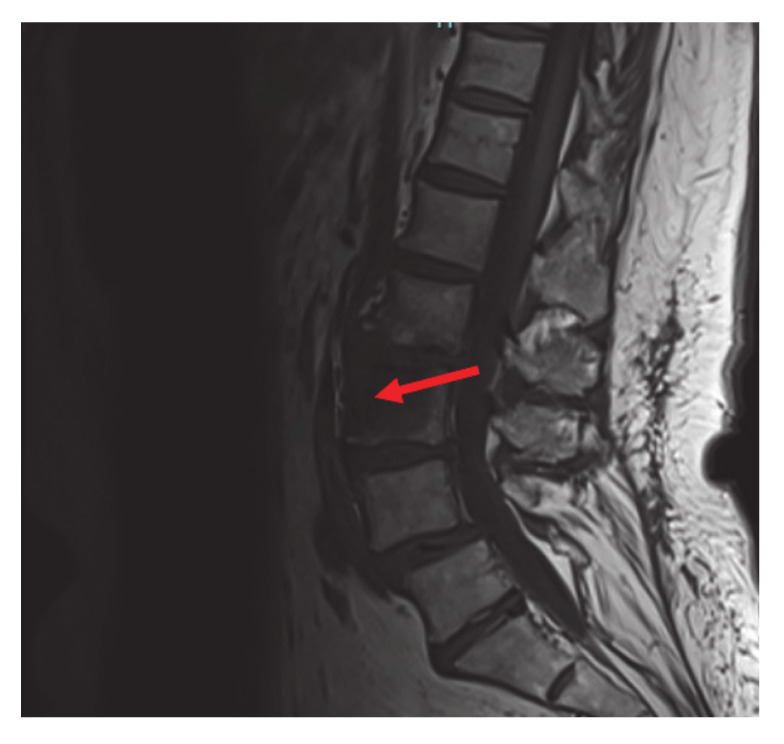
Magnetic Resonance Imaging (MRI) lumbar spine: L3 vertebral body metastasis (red arrow) without compression deformity or canal compromise. Not visualized, severe facet arthropathy with grade 1 anterolisthesis of L4 on L5.
